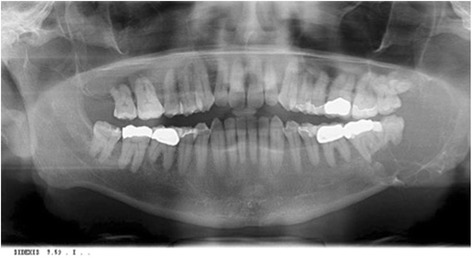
Preoperative panorama X-ray of the jaws with an erosive bone defect of the left mandible
